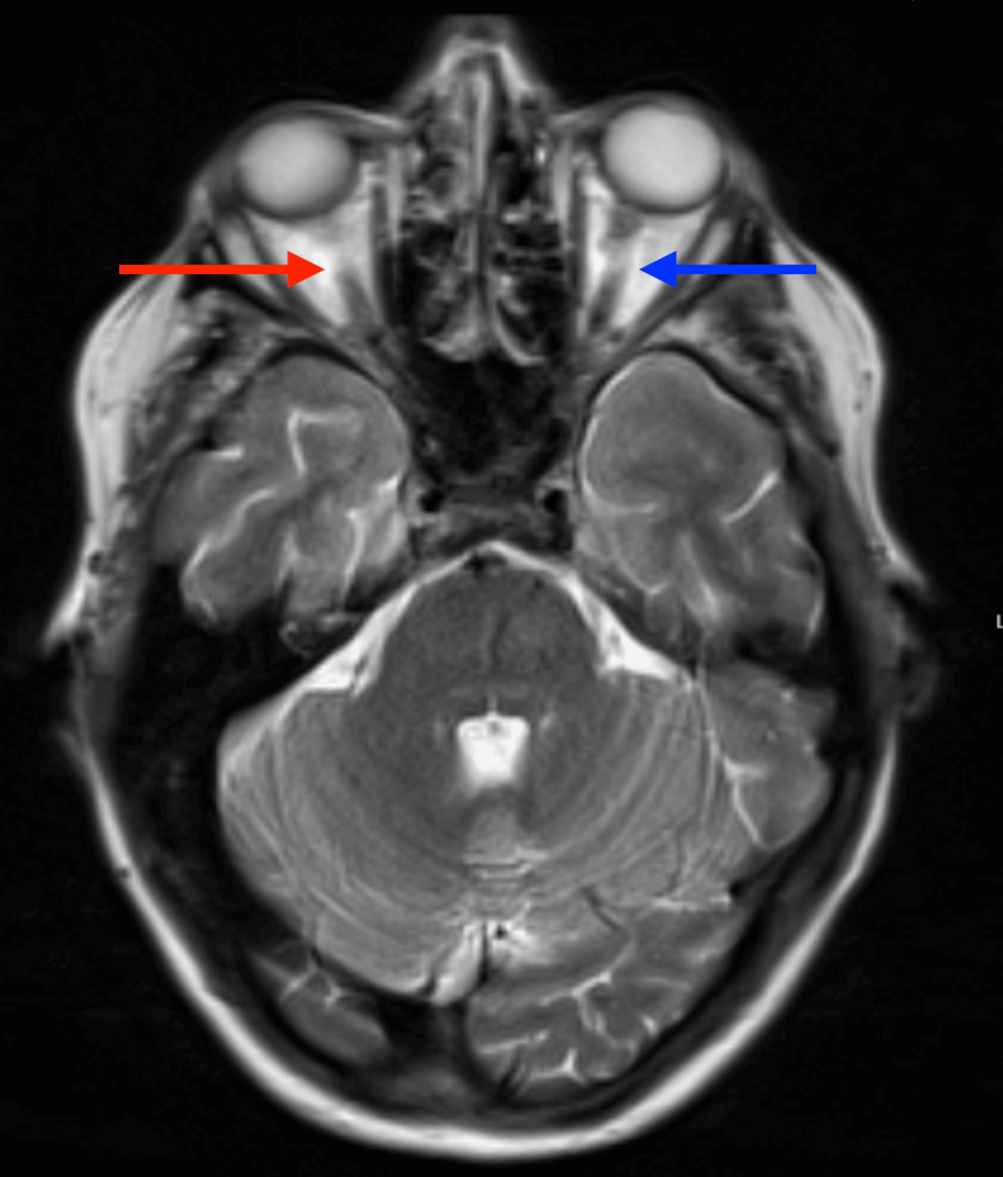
Brain MRI demonstrating prominent superior ophthalmic veins. The right ophthalmic vein (red arrow) is greater in size than the left (blue arrow).
